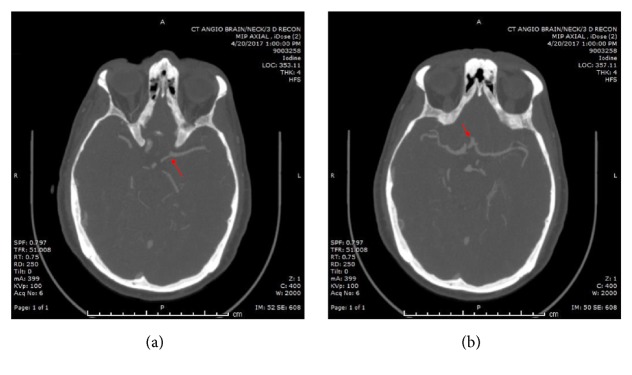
CT angiogram head and neck (continued): note the absence of the left internal carotid artery intracranially and poor opacification of the left ophthalmic artery. There is collateral supply to the left middle cerebral artery from the left posterior cerebral artery (a) and the anterior communicating artery (b). An intact circle of Willis allowed the left middle cerebral artery to maintain supply despite the complete occlusion of the left internal carotid artery.
